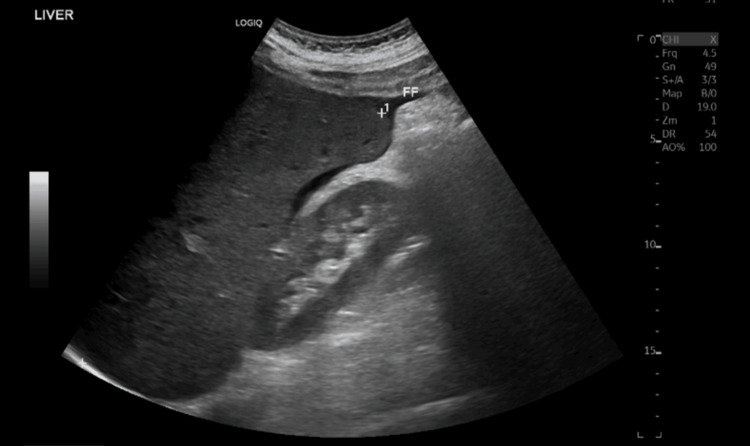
An ultrasound hepatic vein doppler showed hepatomegaly of about 18 cm
